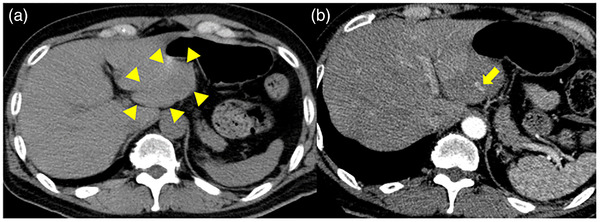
(a) Computed tomography (CT) without intravenous contrast media revealed the presence of hematoma around the stomach (arrowhead). (b) Dynamic CT showed contrast media extravasation from the branch of the left gastric artery (arrow) and hematoma in the lesser omentum
